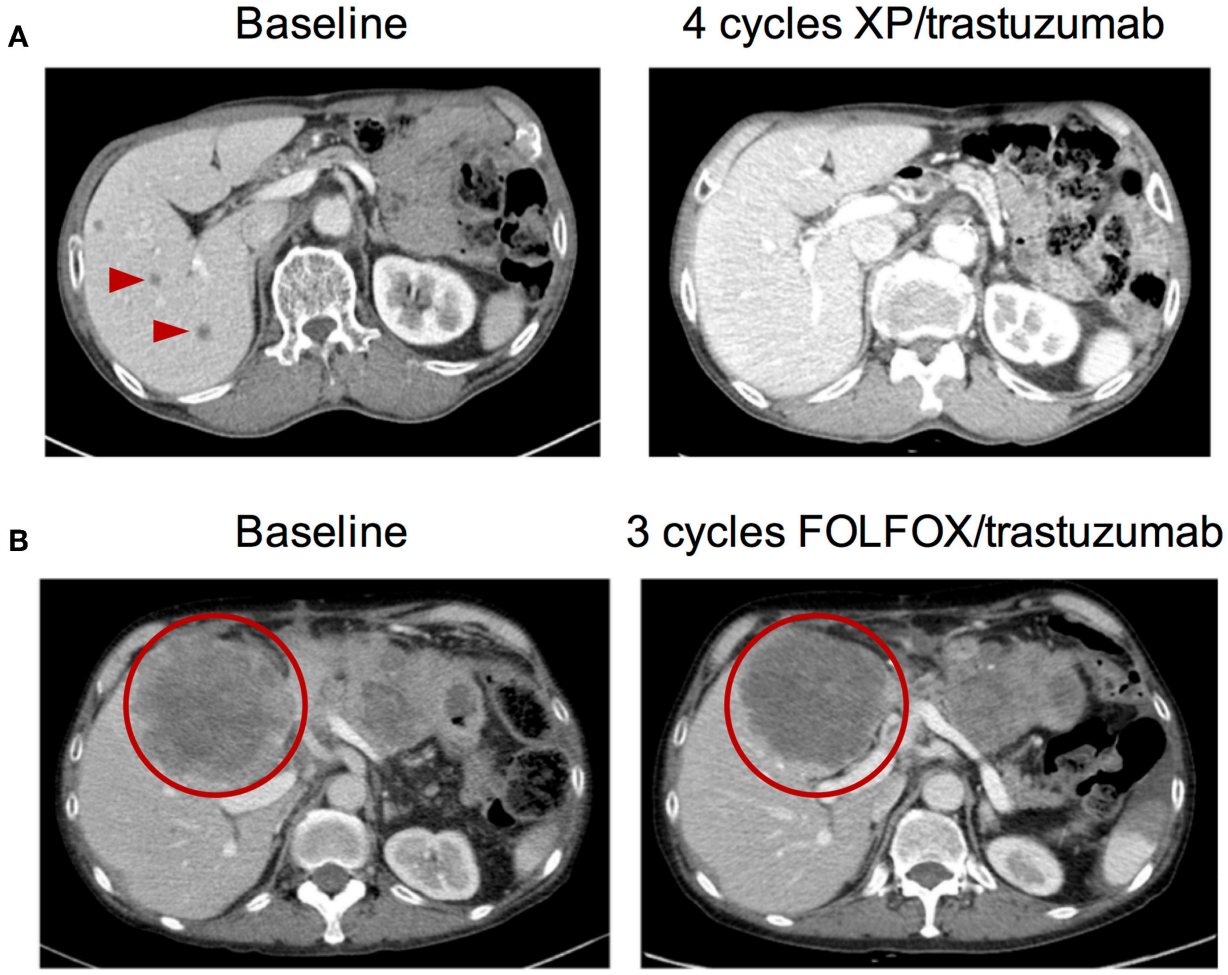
Pre- and post-anti-HER2 therapy abdominal CT images from two *ERBB2*-amplified gastric cancer patients identified by cell-free DNA. (A) Patient #1 at baseline and after four cycles of capecitabine/cisplatin (XP) combined with trastuzumab. (B) Patient #2 at baseline and after three cycles of FOLFOX combined with trastuzumab. Arrowheads and circles denote malignant lesions.
